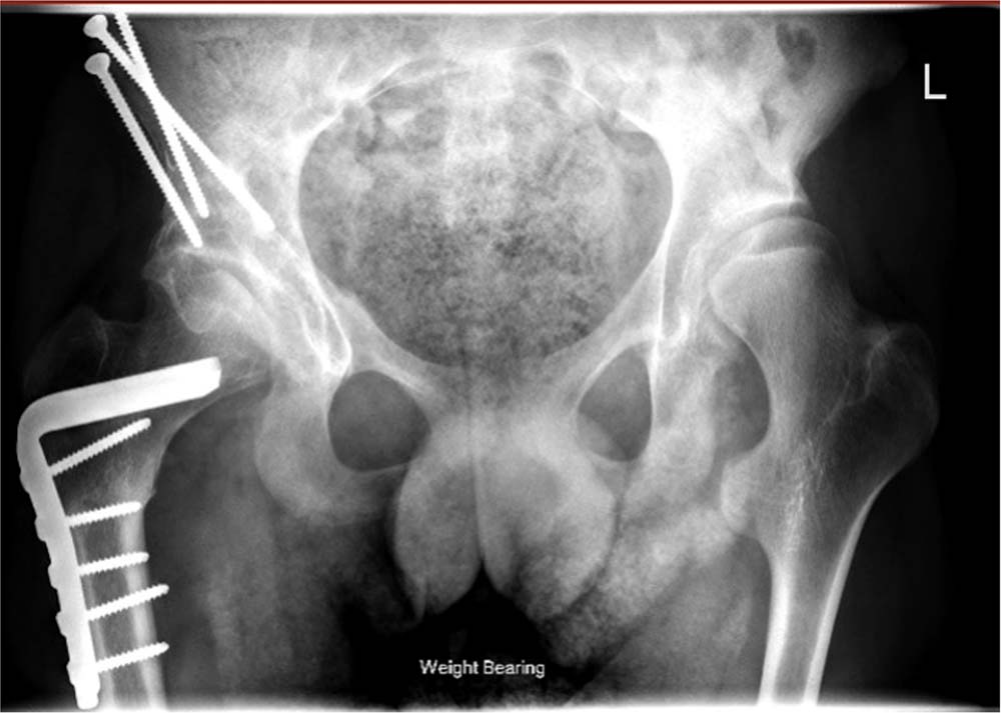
Case 2 post-operative radiograph.
